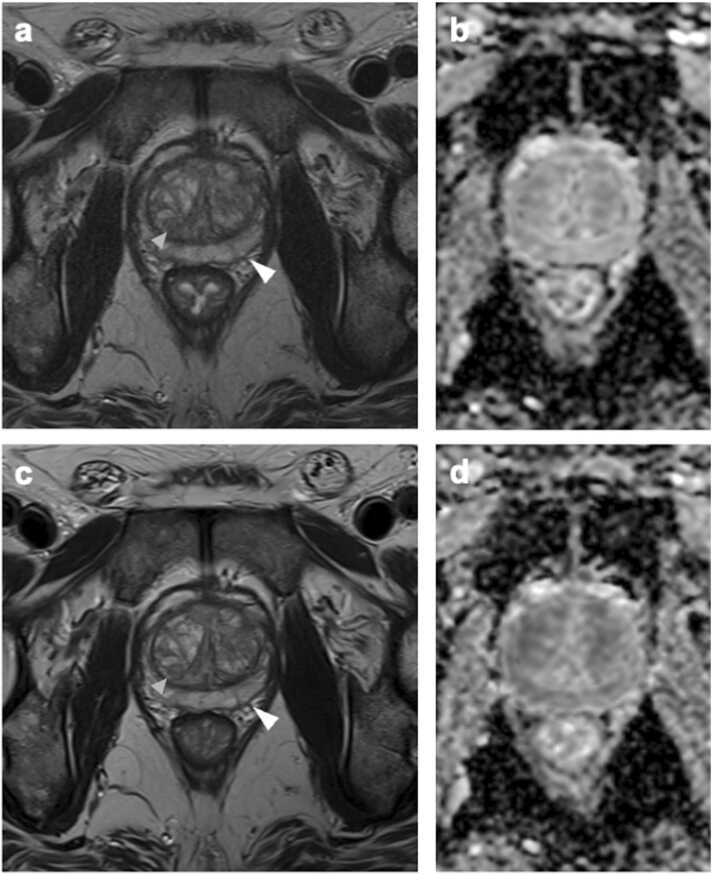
Example for visual comparison of conventional mpMRI (a: T2 TSE, b: DWI) to ultra-fast bpMRI (c: T2 TSE, d: DWI). mpMRI: Slight blurring around prostate capsule (white arrowhead in a) and capsule of hyperplastic nodule in the transition zone (grey arrowhead in a). Acquisition time of full standard protocol: 20 mins 33 sec. ultra-fast bpMRI: Clearer delineation of organ capsule (white arrowhead in c) and capsule of hyperplastic nodule in transition zone (grey arrowhead in c). Acquisition time of full ultra-fast bpMRI: 3 min 28 sec (time savings of approximately −80 %).
